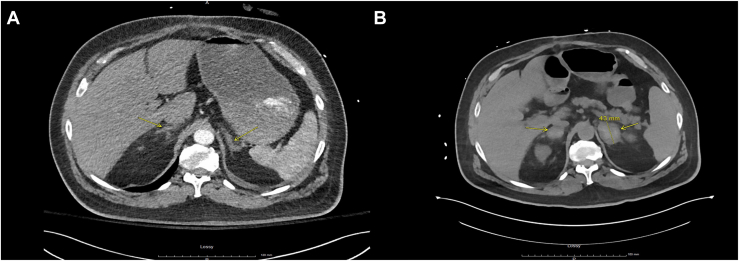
A*, the initial CT abdomen and pelvis showing normal adrenal glands upon initial presentation. *B*, the CT abdomen and pelvis on the night of thrombocytopenia nadir, showing bilateral heterogenous enlarged adrenal glands representing bilateral adrenal hemorrhage. *CT* = computed tomography.
